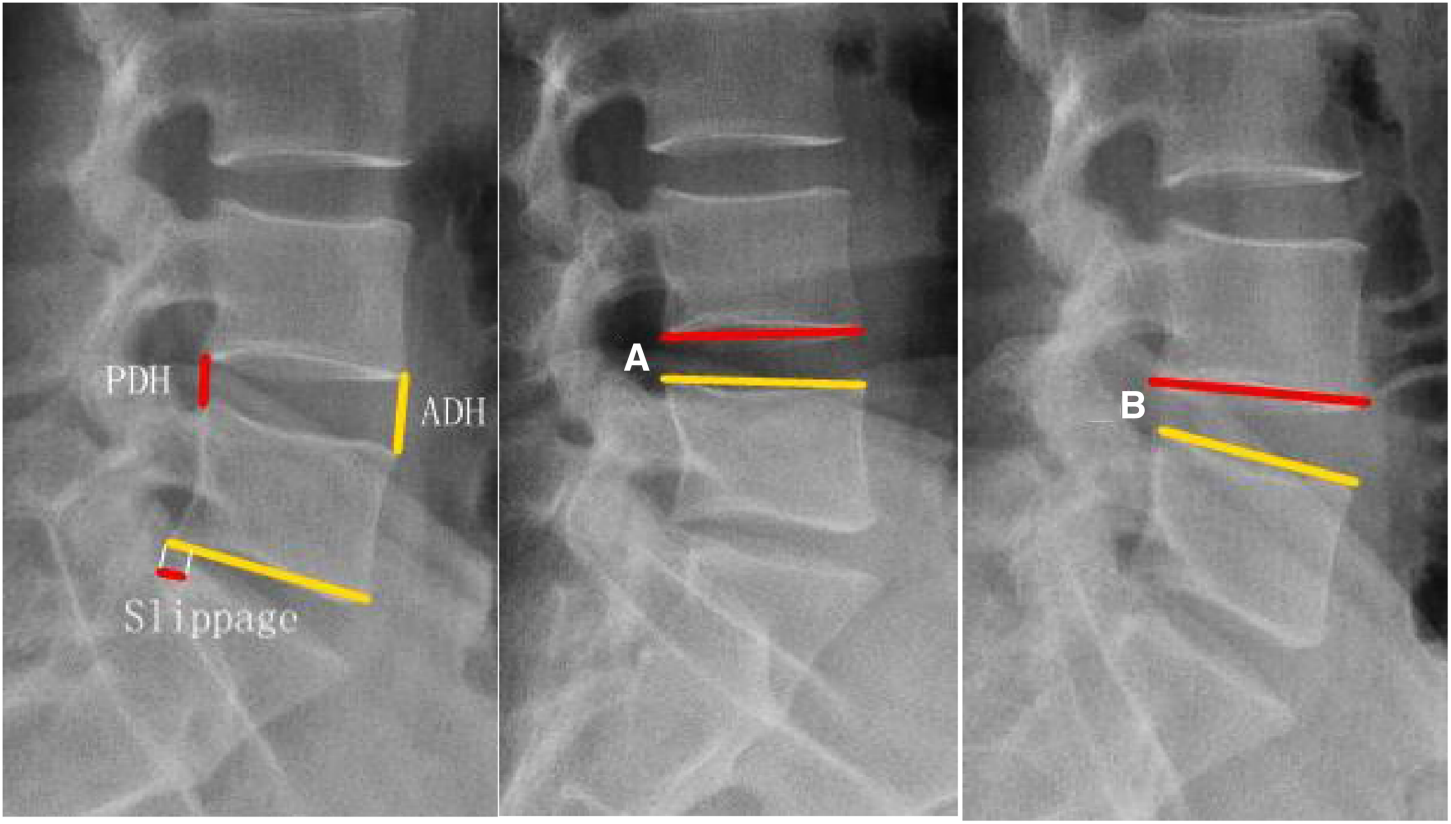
Measurement of radiographic parameters. The IVD space height, posterior disc height (PDH), and anterior disc height (ADH) were obtained and compared. IVD space height = (PDH + ADH)/2; angular motion = Cobb A-Cobb B; translation motion = the length of slippage.
